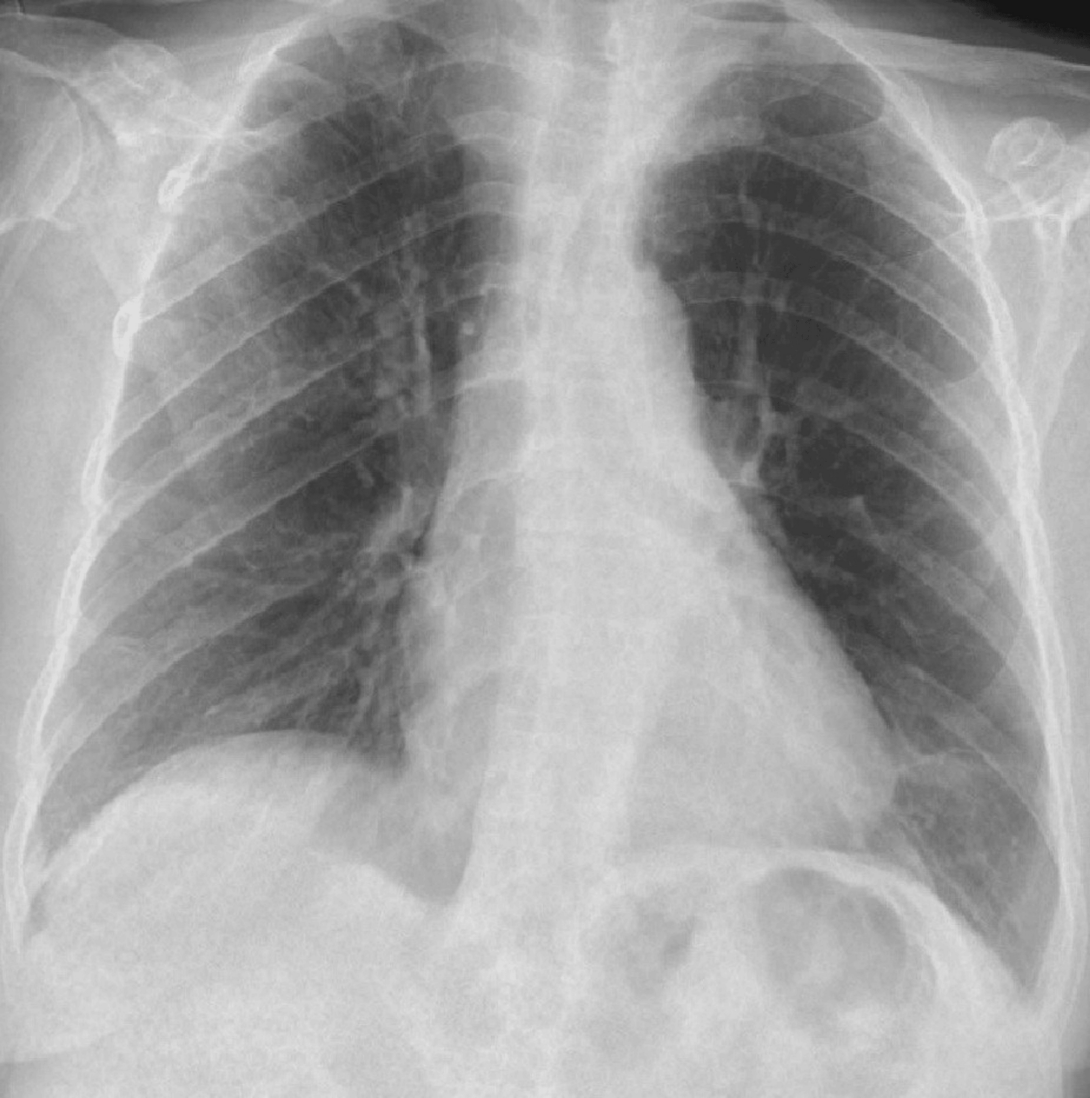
Chest X-ray on the day of admission. Normal chest X-ray showing no significant radiographic abnormalities.
